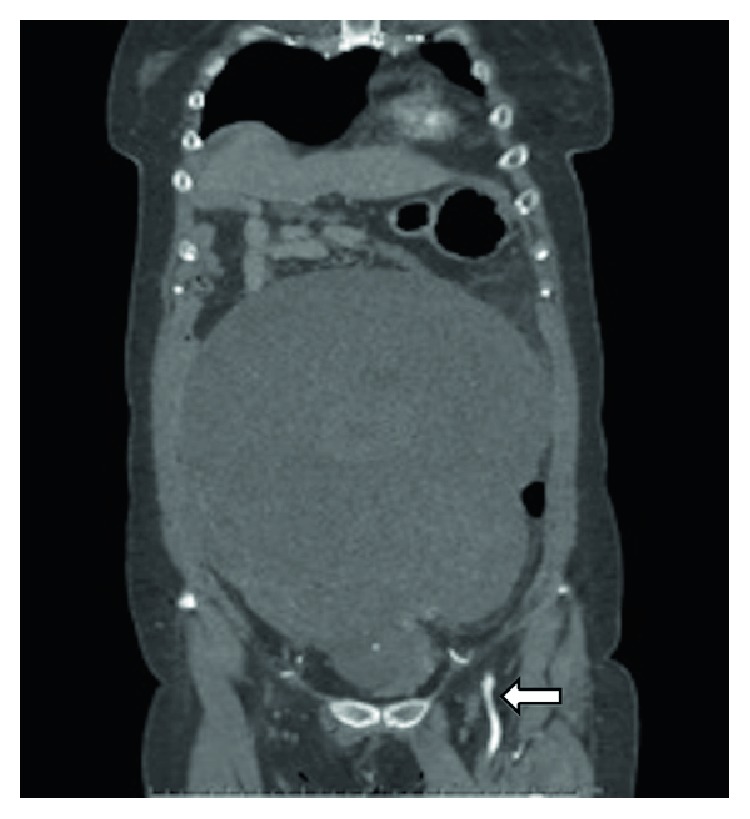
Contrast-enhanced computed tomography. The tumor received its blood supply mainly from the left internal iliac artery (arrow).
